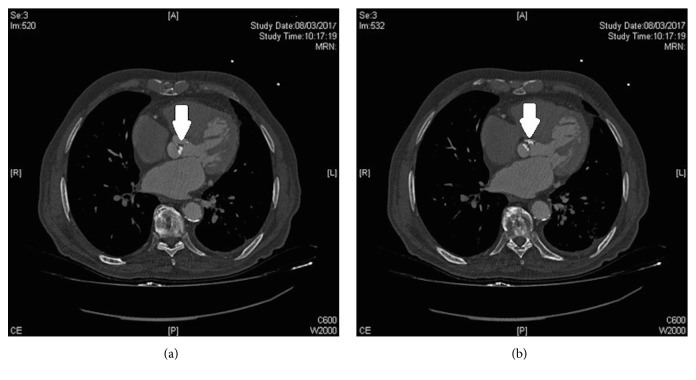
Chest computed tomography scan showing quantification of aortic valve calcification (a and b) (arrows).
